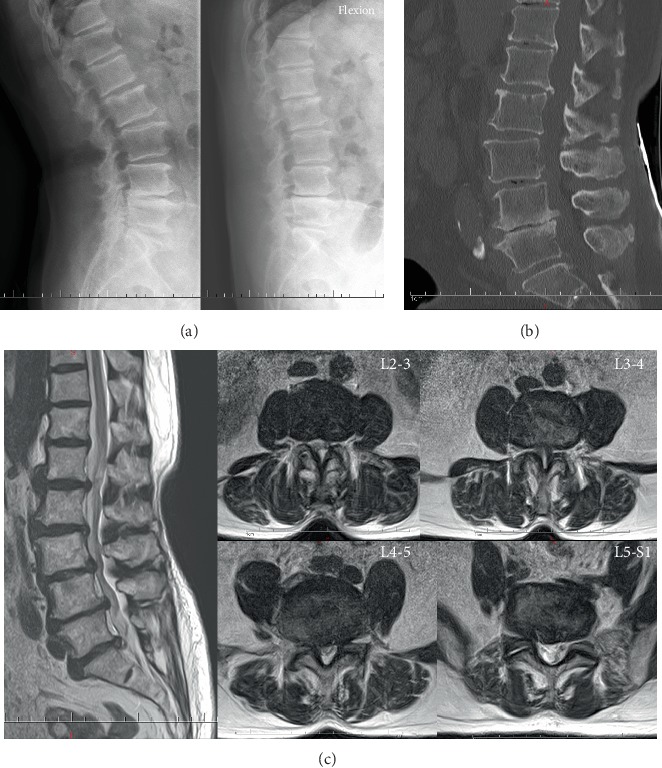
Flexion and extension lateral lumbar X-ray radiograph demonstrating segmental dynamic instability at L2-3 and L3-4 (a). Sagittal lumbar CT showing intervertebral space collapse and osteophyte at L4-5 and “gas sign” at L3-4 and L4-5 discs (b). Sagittal and axial T2-weighted lumbar MRI revealing serious central canal stenosis at L3-4, lumbar disc herniation and right lateral recess stenosis at L4-5, and moderate canal stenosis in L2-3 (c).
